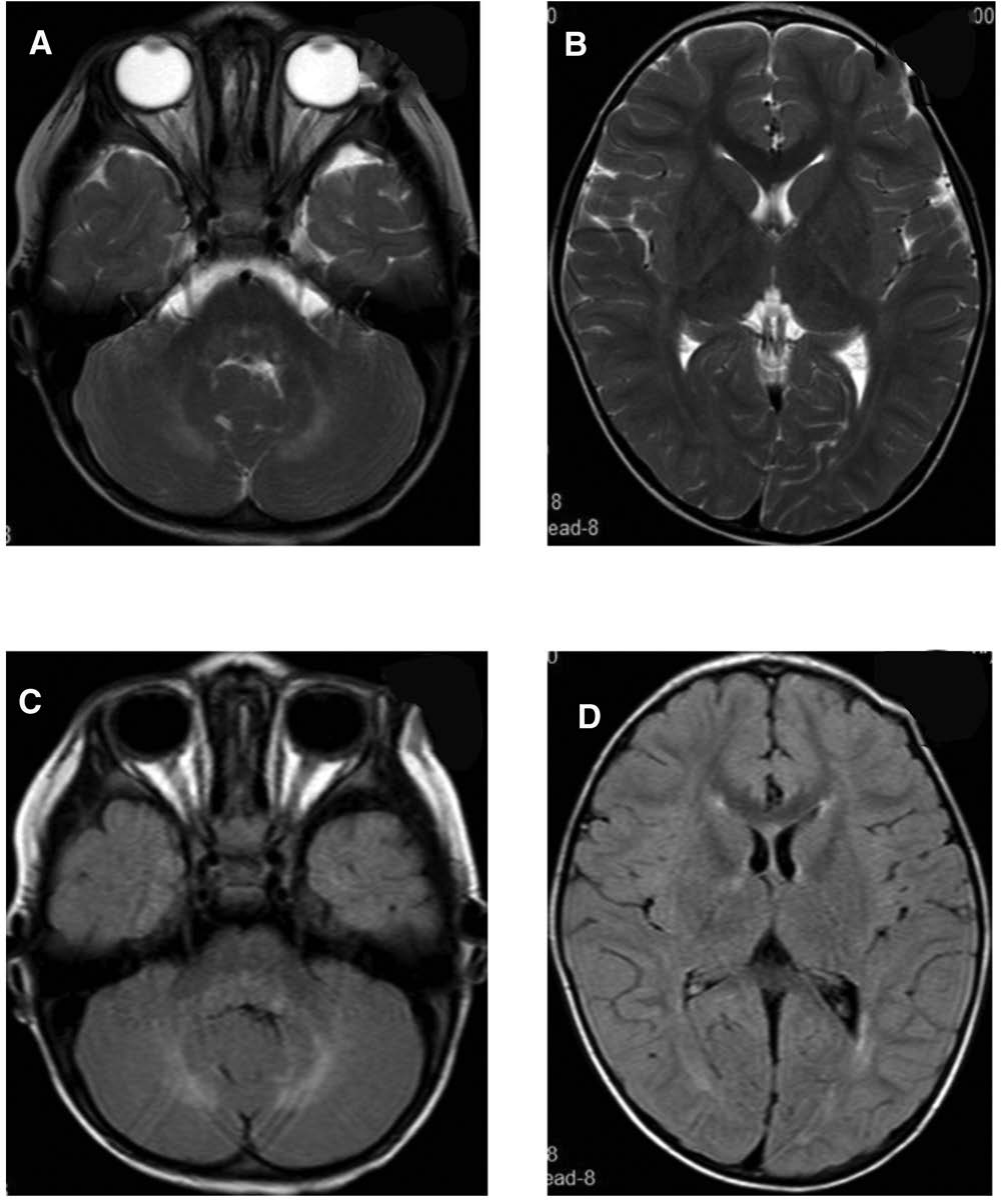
Cranial MRI of Case 1 at the age of 2 years and 8 months. Multiple patchy, slightly long T2 and FLAIR high signal shadows found at white matters of bilateral frontal, parietal, and occipital lobes, as well as basal ganglia, bilateral cerebellar hemispheres, and dorsal pons, with unclear boundaries. The posterior parts of the bodies of bilateral lateral ventricles were slightly widened. FLAIR = fluid-attenuated inversion recovery, MRI = magnetic resonance imaging.
